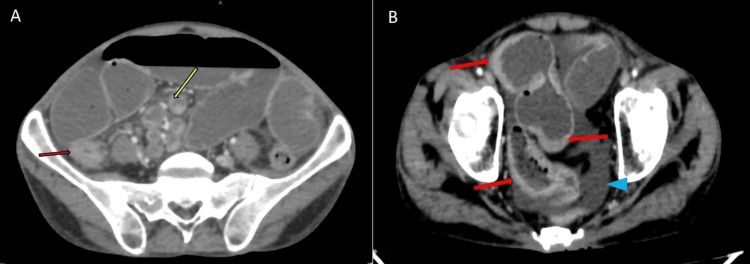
Contrast computed tomography scan of the abdomen, axial views. Image A: A contrast computed tomography scan axial image showing abdominal lymphadenopathy (yellow arrow) and small bowel stricture (red arrow). Image B: Contrast computed tomography scan axial image showing long segment circumferential bowel wall thickening with luminal narrowing suggesting small bowel stricture (red arrows) and ascites (blue arrowhead).
